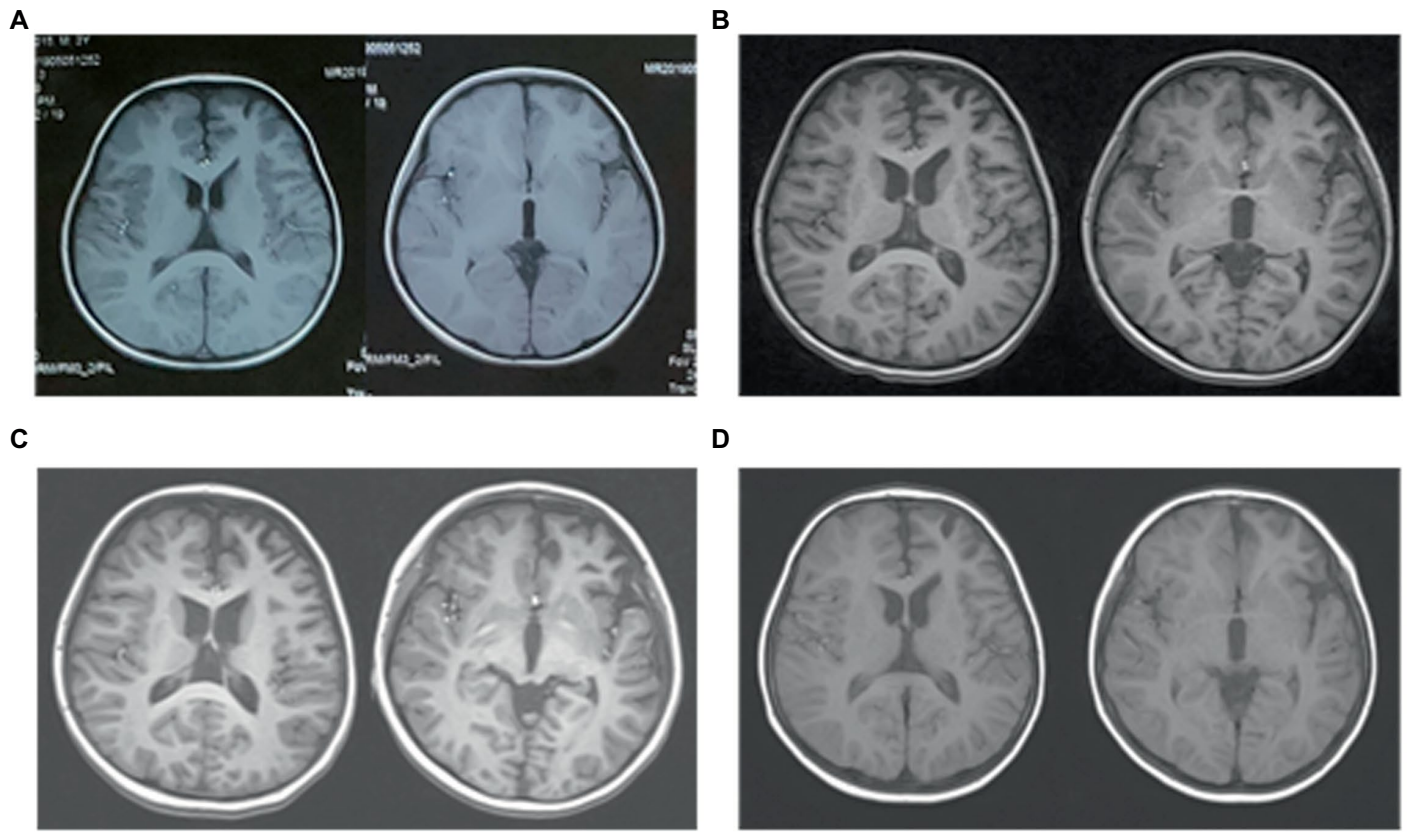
Brain MRI of case 2. (A) Three months before admission, axial T1WI shows slightly deeper sulci in left frontal lobe. (B) On admission, axial T1WI shows mild atrophy of the left hemisphere, especially frontal and temporal lobe. (C) Three months after immunotherapy, there are no obvious progression of cerebral atrophy. (D) Follow-up imaging at 9 months after immunotherapy identifies no further damage.
